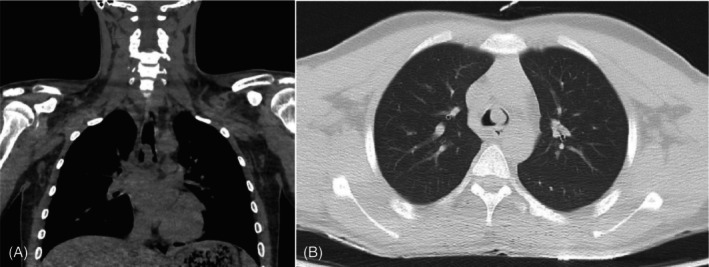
Chest computed tomography scan. Coronal (A) and axial (B) image a pedunculated polypoid mass measuring approximately 11 × 13 mm in cross‐sectional diameter, situated in the distal trachea and extending over a cranio‐caudal length of 21 mm.
